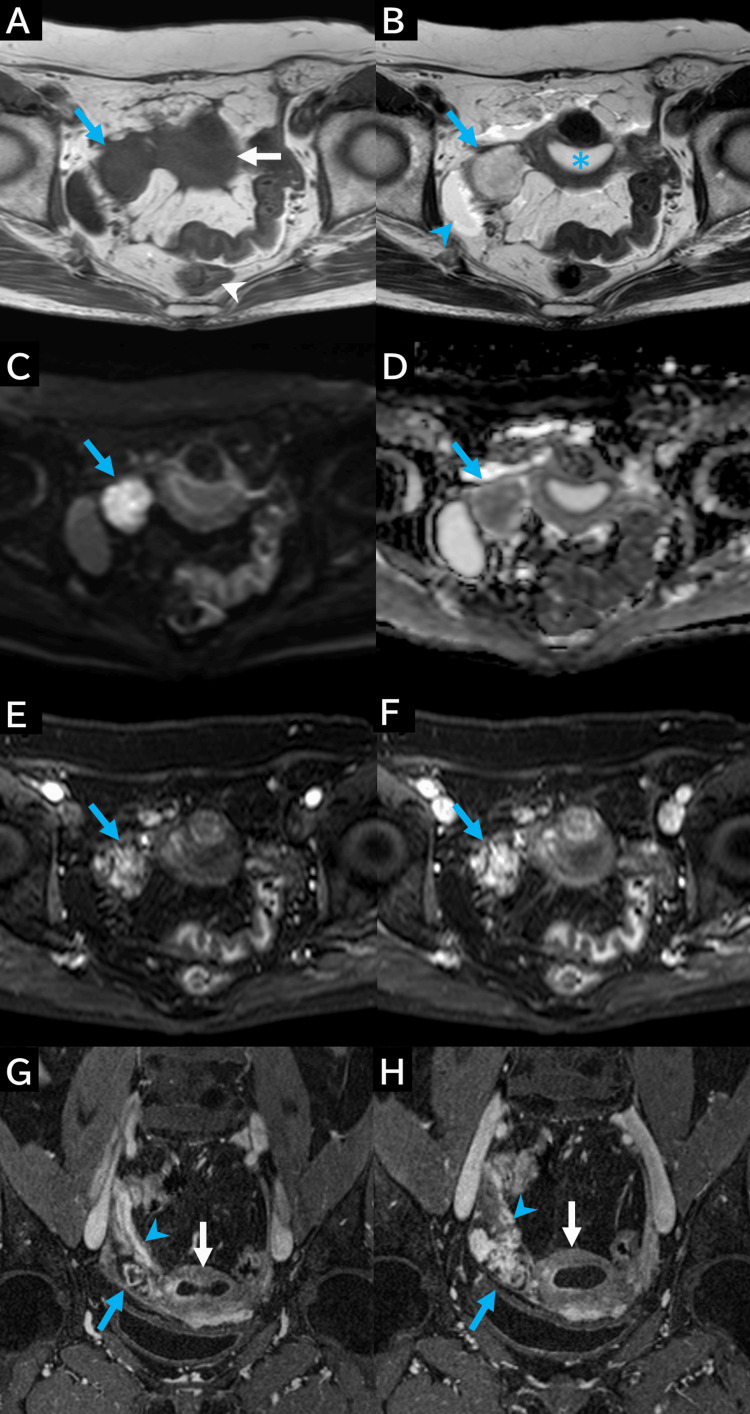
MRI findings (A) In contrast to CT findings, the mass is on the right side of the uterus (white arrow) and is separated from the rectum. On T1-weighted imaging, the mass (blue arrow) demonstrates signal intensity similar to that of the myometrium. (B) On axial T2-weighted imaging, the mass (blue arrow) shows heterogeneous high signal intensity with a low-signal-intensity rim, indicating a high-water content. Small amounts of fluid are observed around the mass (blue arrowhead) and within the endometrial cavity (blue asterisk). These findings are uncommon in postmenopausal women and suggest a fallopian tube lesion. (C) Axial diffusion-weighted image reveals a high-signal-intensity mass (blue arrow). (D) On the ADC map, the mass shows heterogeneous low signal intensity, suggesting a high likelihood of malignancy (blue arrow). (E) An early-phase (35 seconds) contrast-enhanced axial fat-saturated T1-weighted image reveals high signal intensity within the mass compared to the myometrium (blue arrow). (F) A delayed-phase (125 seconds) contrast-enhanced axial fat-saturated T1-weighted image reveals strong patchy high signal intensity within the mass (blue arrow). (G and H) A delayed-phase (180 seconds) coronal contrast-enhanced fat-saturated T1-weighted image reveals strong patchy high signal intensity within the mass (blue arrow). The right ovarian vein runs along the internal surface of the right adnexal tumor (blue arrowhead). The right ovarian vein (blue arrowhead) is continuous with the tumor on the right side of the uterus (white arrow). Although the tumor appears to be in a different location compared to that on the CT images, its continuity with the right ovarian vein and similar internal characteristics suggest that it is the same lesion. Fluid is present in the endometrial cavity (white arrow), which is uncommon in postmenopausal women and further supports the diagnosis of a fallopian tube lesion. ADC: apparent diffusion coefficient; MRI: magnetic resonance imaging
